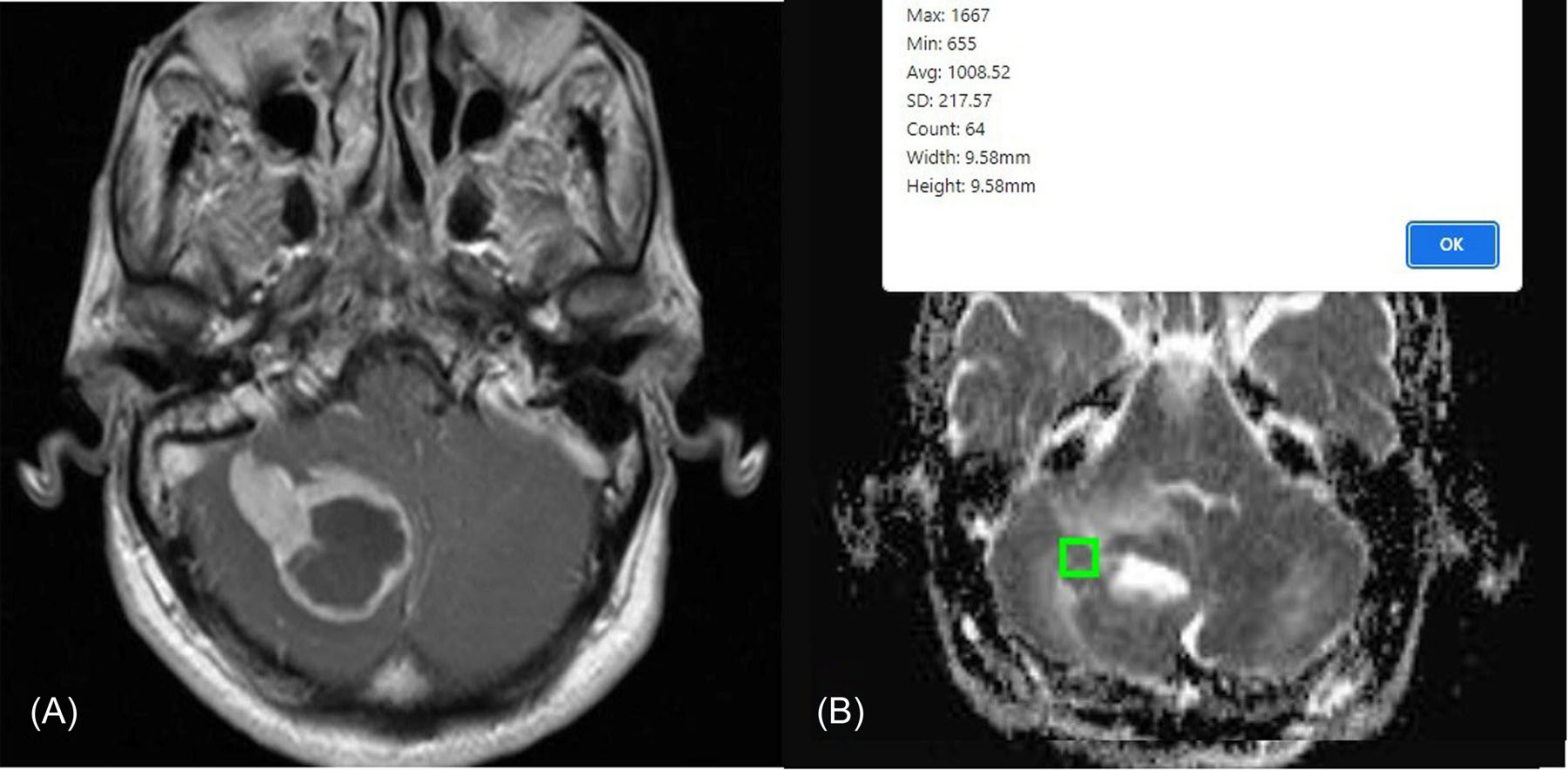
(A) Contrast‐enhanced axial T1‐weighted MRI of a brain tumor for identifying the tumor's location and its components. (B) measuring the apparent diffusion coefficient (ADC) value by placing region of interest (ROI) on the darkest point of the solid section of the tumor in the ADC sequence.
